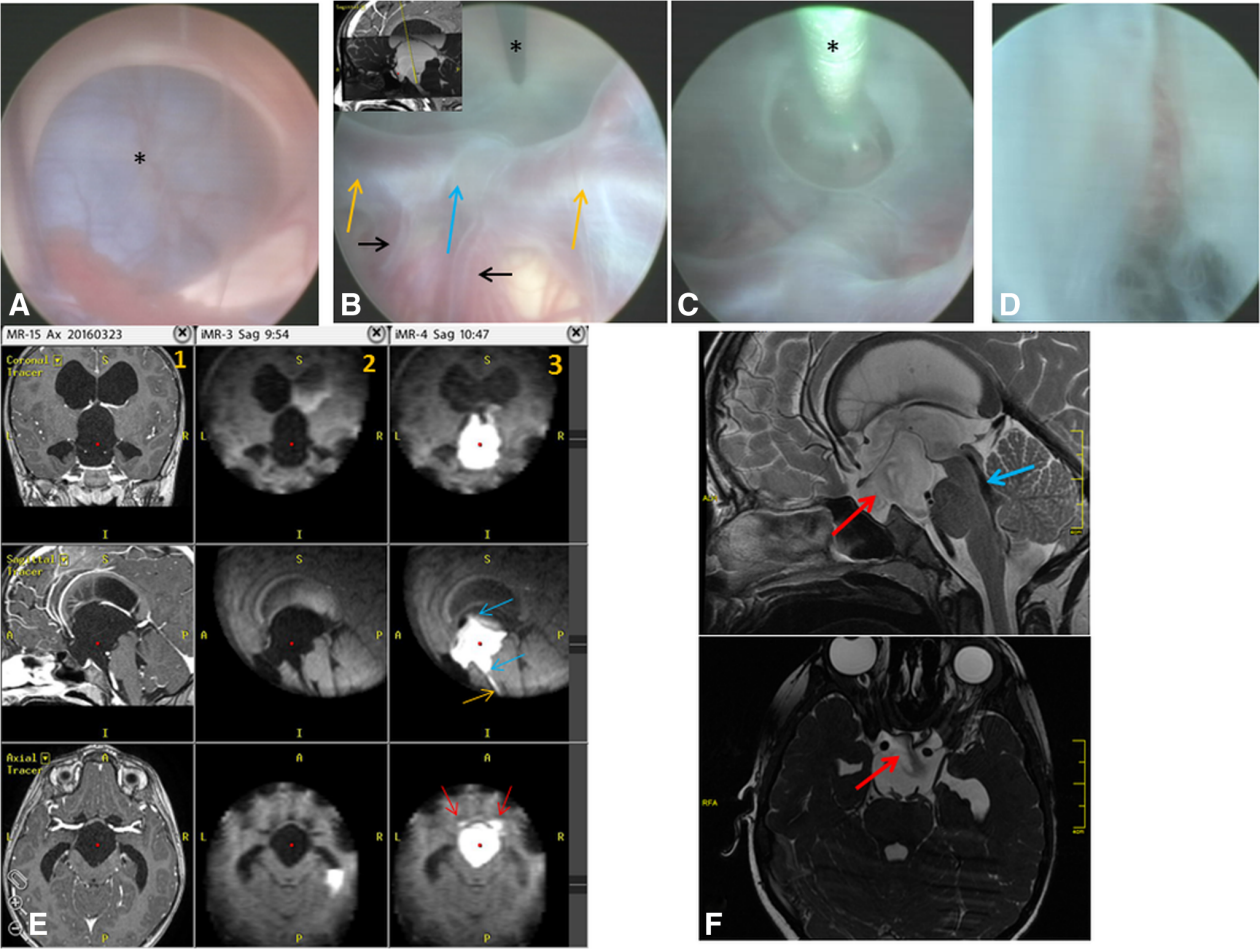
Images demonstrating the endoscopic procedure performed in a 8-year-old boy (patient 14) suffering from HCP and pituitary insufficiency due to a large suprasellar cyst. a Endoscopic view of the right lateral ventricle occluded by an arachnoid cyst bulging through the foramen of Monroe (asterisk). b View of the cyst floor after the performance of endoscopic perforation of the upper cyst wall at the level of foramen of Monro. MRI T2-weighted scan in a sagittal plane, presented in the left upper corner, is showing the plan for bilateral cyst perforation. Note how deep the cyst penetrates the space between the brain stem and clivus. The basilar artery tip (blue arrow), together with both posterior arteries (yellow arrows) and their deep branches (black arrows), are located relatively high to the site planned for cystostomy pointed by a monopolar probe (asterisk). c The 3F Fogarthy balloon catheter (asterisk) is enlarging the fenestration in the narrow space between the trunk of the basilar artery and clivus. d Final view of the fenestration. e MRI scans of the arachnoid cyst before its fenestration (column 1—high-field 1.5-T images; column 2—low-field intraoperative 0.15-T images after contrast administration into the right lateral ventricle) and after the cystoventriculostomy (column 3). The cyst initially was totally separated from the arachnoid and ventricular system blocking the cerebrospinal fluid flow through the third ventricle towards the aqueduct (columns 1 and 2). After the bilateral cyst fenestration (column 3, blue arrows), the contrast traverses the cyst and flows to the arachnoid space of the prepontine area (yellow arrow). The cyst has shrinked in comparison with its preoperative shape. Red arrows show the distal contrast flow reaching the Sylvian fissure arachnoid space. f Postoperative high-field sagittal T2-weighted and axial heavily T2-weighted steady-state (3D-DRIVE) MRI scans performed 1 year after the surgery. Note the evident shrinkage of the cyst volume enabling a free flow of CSF through the third ventricle. Red arrows show the flow void phenomenon through the fenestrated cyst while the blue one the flow void through the Sylvian aqueduct
